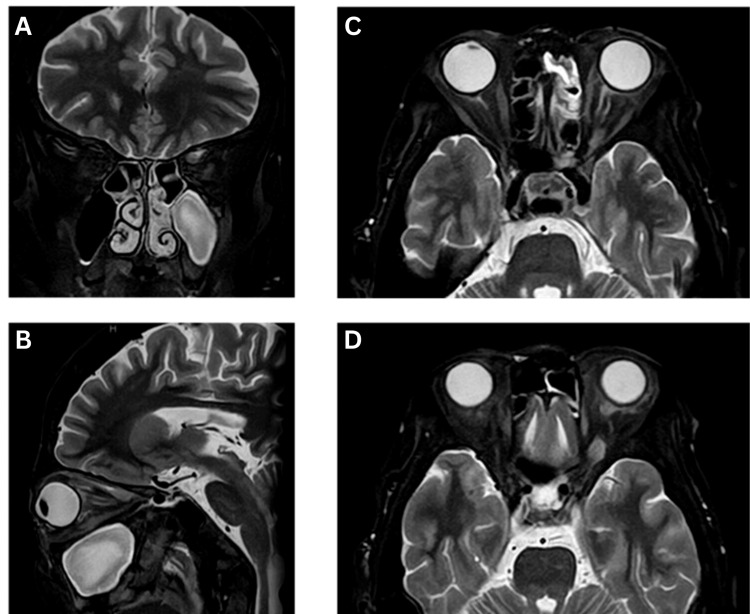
Orbital MRI images before treatment (A) Coronal section, (B) sagittal section, and (C-D) horizontal section. An irregular lesion was found in the muscle belly of the left superior rectus muscle. MRI: magnetic resonance imaging
